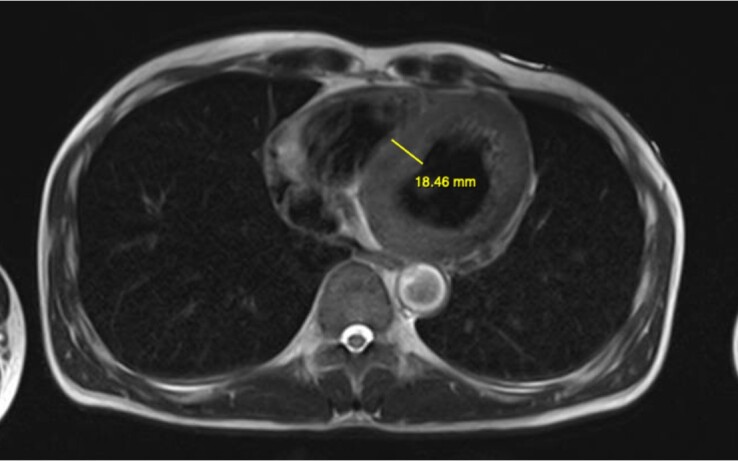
Cardiac MRI showing myocardial edema with the left ventricular wall thickening (8-18 mm) in axial T2-weighted image.
